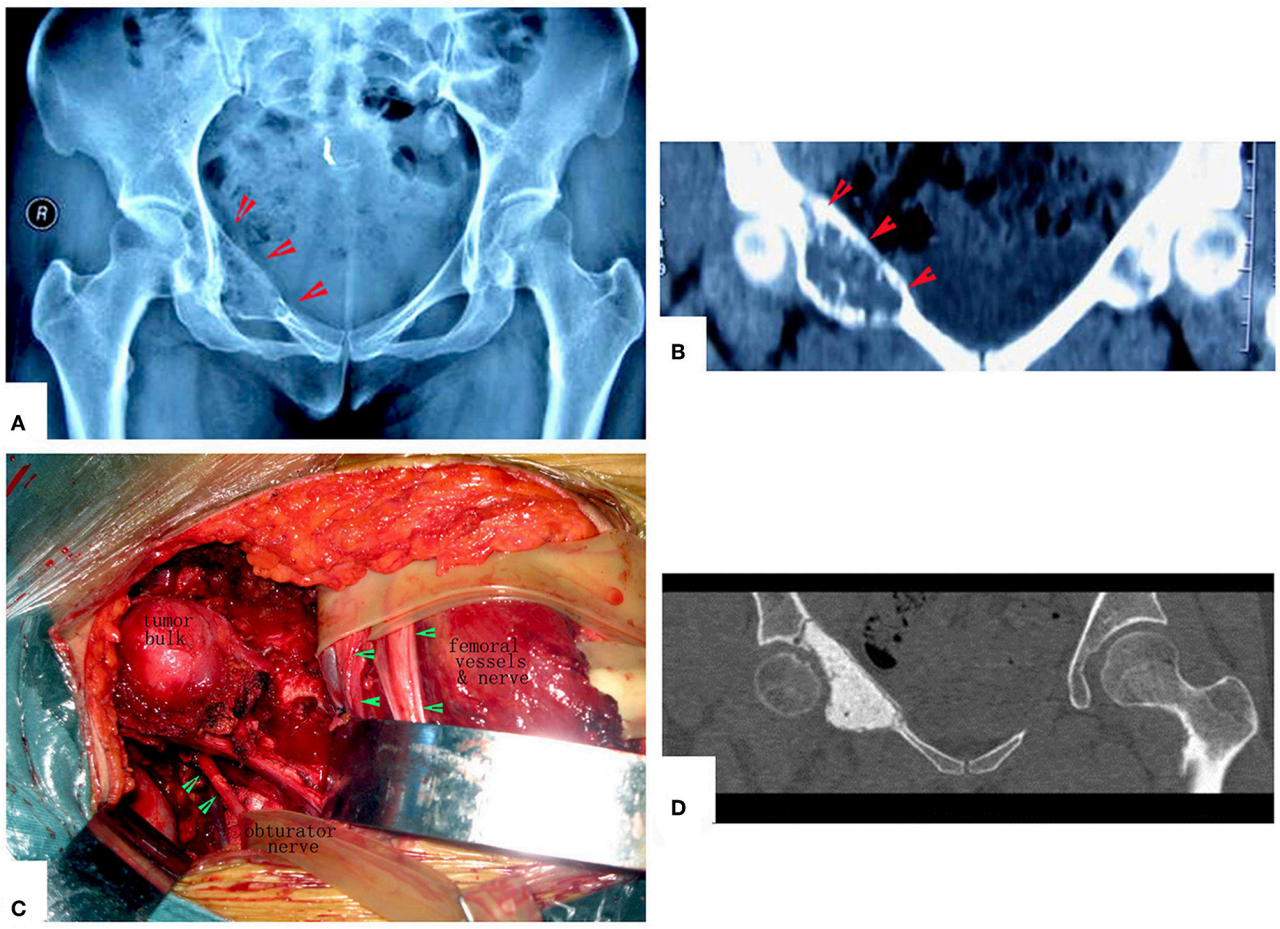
Management of periacetabular chondrosarcoma. (A,B) X-ray film and CT scan. Anteroposterior radiograph of the pelvis showing a periacetabular chondrosarcoma on the right. CT of the pelvis, showing the destruction of the inner part of the upper pubic bone. (C) Exposure of the tumor. (D) Images after the operation. The hip joint reserved well. The function is perfect at 8 years after operation.
